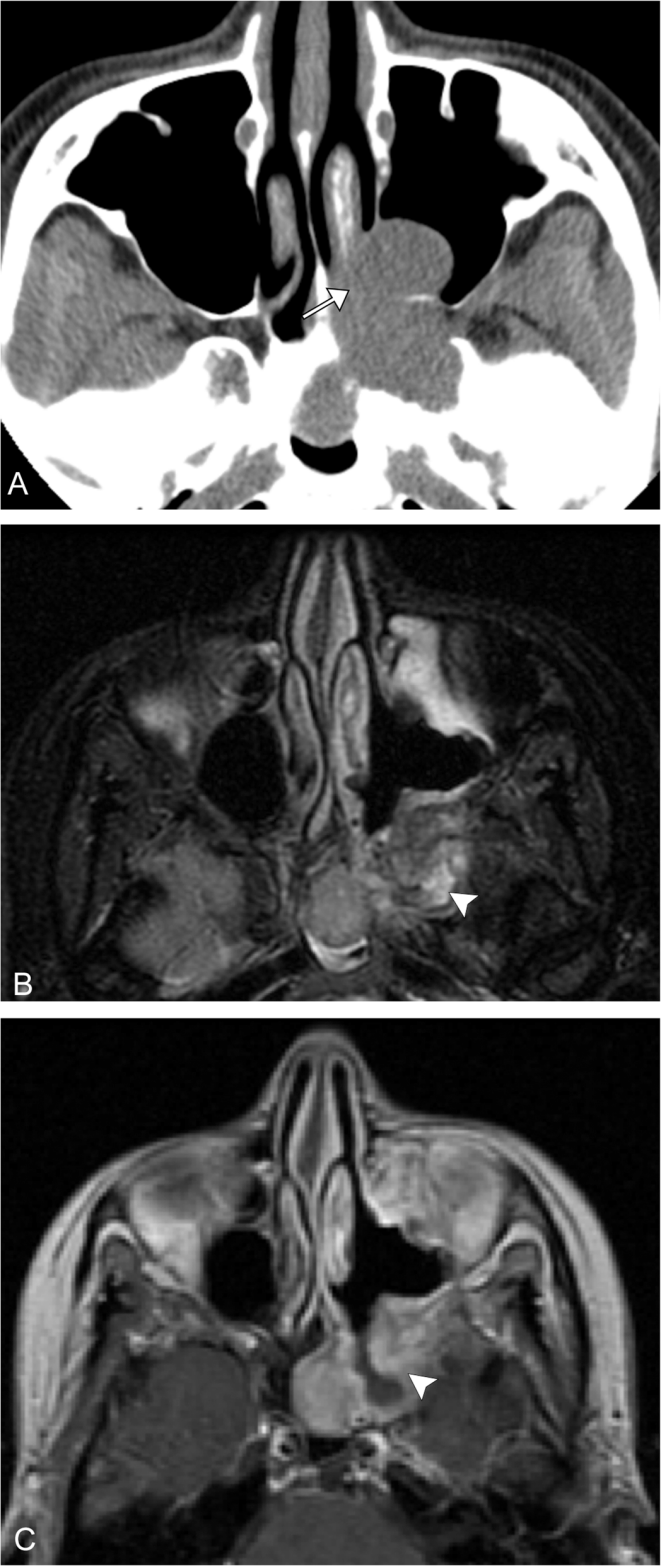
Epithelioid hemangioendothelioma. A 37-year-old female with left facial fullness and recurrent sinusitis. a Axial CT image shows an expansile polypoid lesion centered in the left pterygopalatine fossa, extending into the maxillary sinus and sphenoid sinus, as well as the left nasal cavity (arrow). b Axial T2 image after diagnostic and therapeutic resection demonstrates residual ill-defined mildly T2 hyperintense tissue along the peripheral margins of resection (arrowhead). c Corresponding axial T1 post-contrast image demonstrates avid enhancement of the residual lesion (arrowhead)
